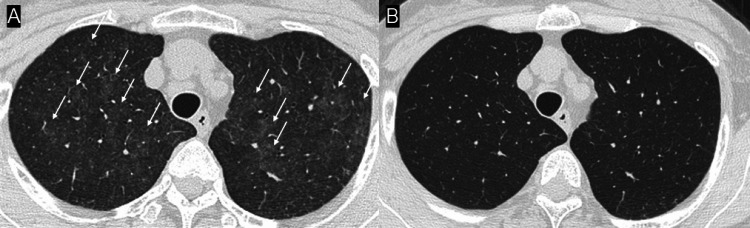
High-resolution computed tomography (HRCT) findings before and after corticosteroid therapy HRCT images showing diffuse centrilobular ground-glass nodules predominantly in the upper lobes (arrows), which markedly improved after corticosteroid therapy (prednisolone 50 mg/day). (A) On admission. (B) Two weeks after initiation of corticosteroid therapy.
